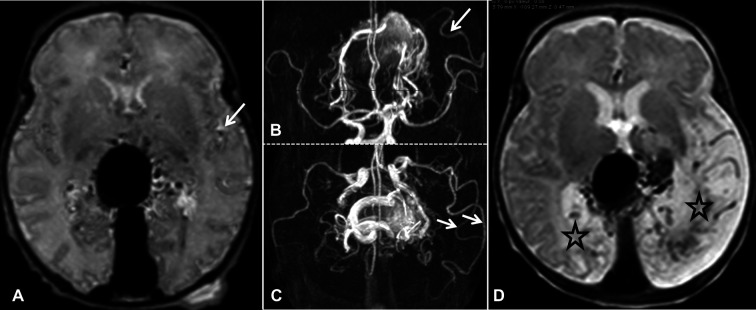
MRI at birth in axial T2-weighted SE (A) and TOF in axial and coronal reformat (B,C, respectively). The brain parenchyma is Normal. However, abnormal dilations of the distal branch of the left MCA are identified (B,C, indicated by arrows). These dilated arteries are also visible in the Sylvian fissure on T2 SE (A, indicated by arrow) and correspond to so-called “pseudo-feeders”. Follow-up SE T2 axial MRI performed 19 days after birth (D). We observe bilateral encephalomalacia, especially in the brain parenchyma, downstream of the dilated pseudo-feeders in the left parieto-occipital lobe (D, indicated by stars).
